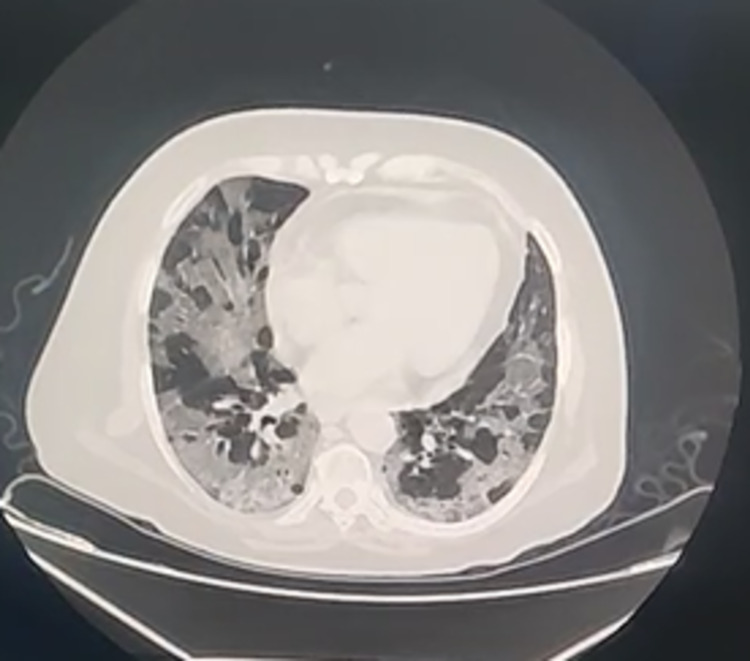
Computed tomography (CT) findings in recovered COVID-19 patient
